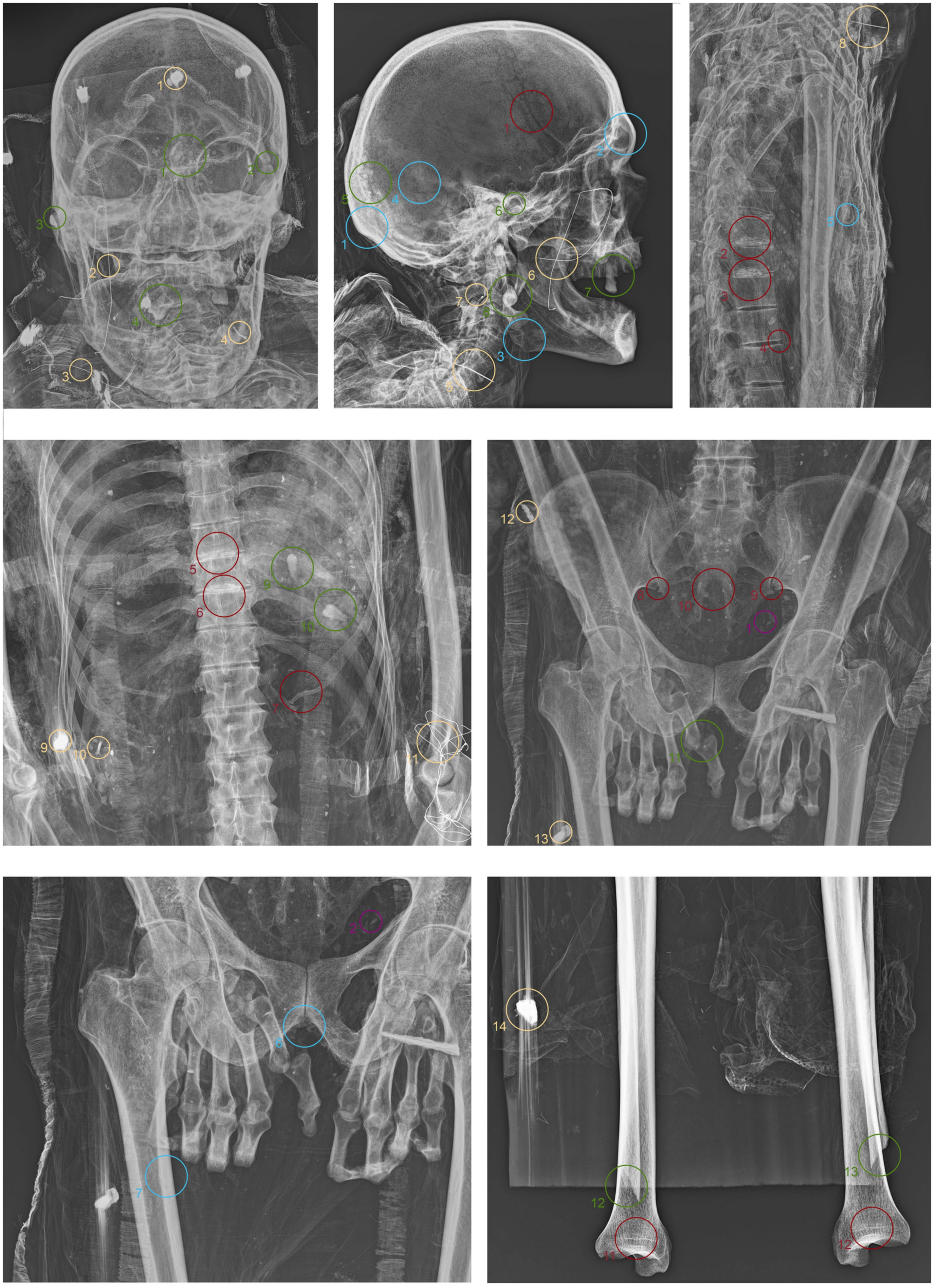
Anteroposterior and lateral radiographic acquisitions of the individual ID1. Green highlights indicate areas of interest for taphonomic study, yellow highlights indicate areas relevant to the assessment of restorations predating this study, violet is used for entomological analysis, blue for anthropological aspects, and red for paleopathological evidence.
